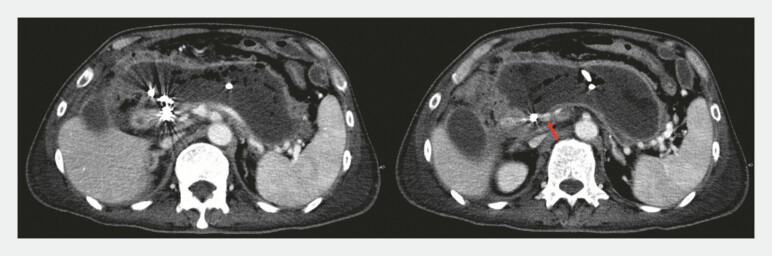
Computed tomography images taken 42 days after transcatheter arterial embolization for a pseudoaneurysm of the gastroduodenal artery inside the walled-off necrosis (WON) showing significant amounts of intra-WON necrotic remnants and blood flow (red arrow) just adjacent to the embolization coils in the wall of the WON.
